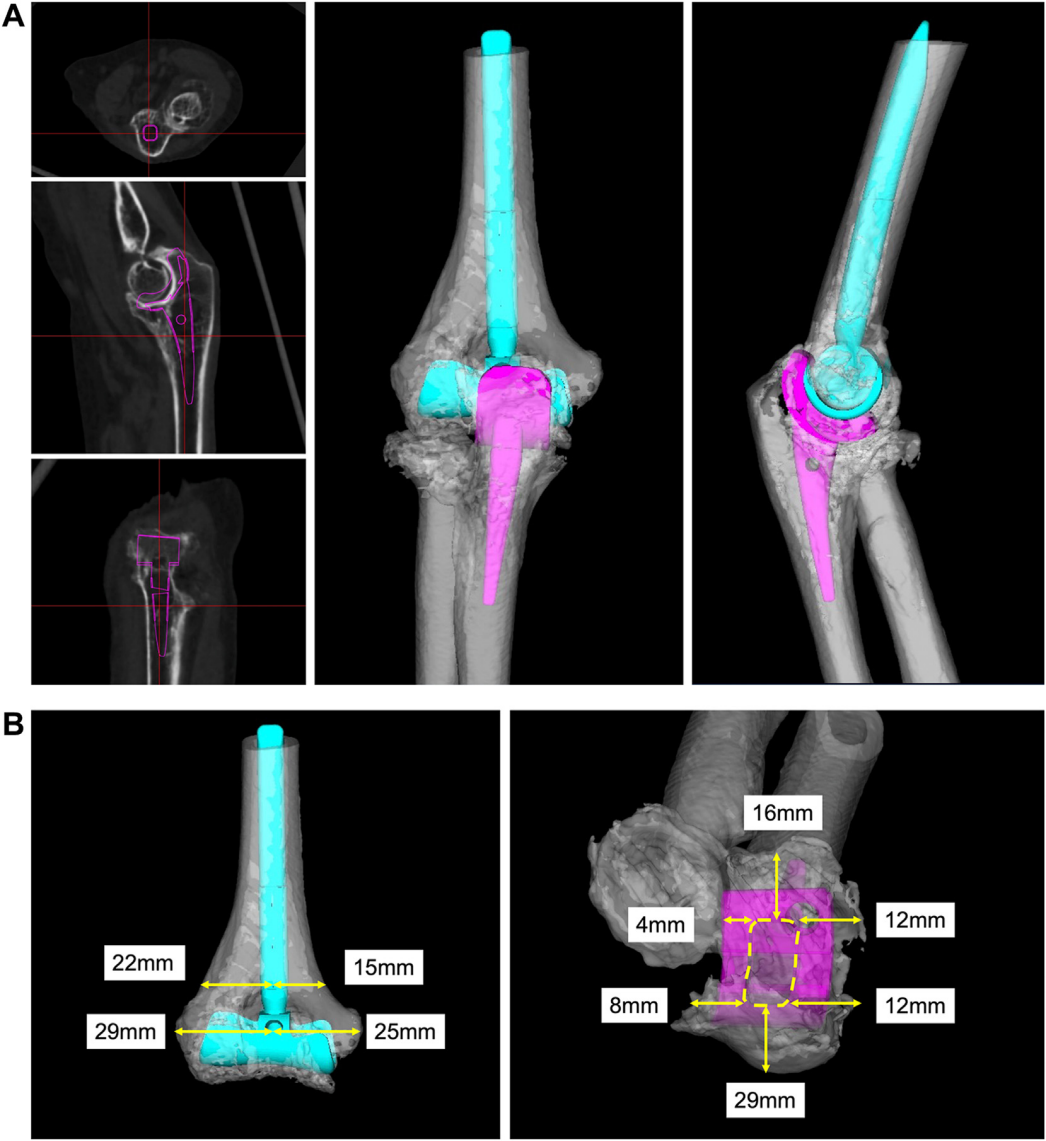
Computed tomograhy-based 3D preoperative planning for TEA. (A) The implant size and position are determined to fit the humeral and ulnar canals in the coronal, sagittal, and axial views (Left). Humeral component rotation is positioned parallel to the humeral epicondylar axis. Ulnar component rotation is oriented parallel to the surface of the humeral component. (B) To reproduce the planned placement position during surgery, several parameters around the bone tunnel are determined, and these measurements are used as a reference for implant placement during surgery. *3D*, 3-dimensional; *TEA*, total elbow arthroplasty.
